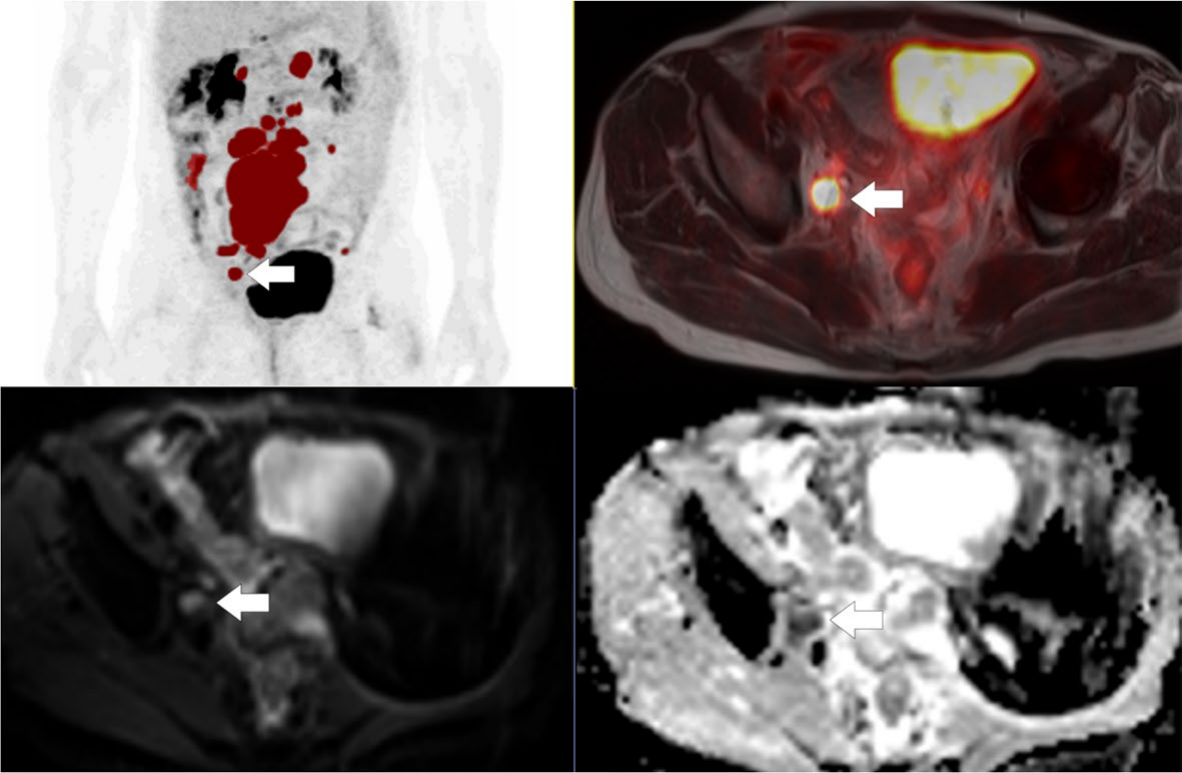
Baseline PET/MRI of a73-year-old male with Diffuse Large B-cell Lymphoma, stage IIAX, IPI 3 in progression at end of treatment scan. Metabolic tumor volume (MTV) was 375 cm^3^. The region of interest was a 16x11mm FDG avid target lesion in right pelvic/iliac region. Minimum apparent diffusion coefficient (ADC_min_) was 365 mm^2^/s and mean apparent diffusion coefficient (ADC_mean_) was 770 mm^2^/s. Upper left: PET MIP MTV. Upper right: Fused PET with T2-HASTE. Lower left: Diffusion weighted imaging (b800). Lower right: ADC-map
